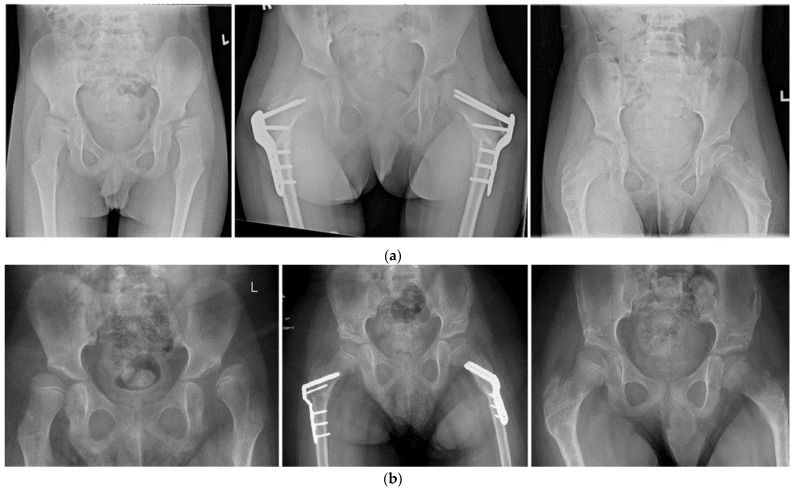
Case examples of hip reconstruction. (a) Case 1: Pediatric Hip Plate. Patient 1 is a boy with CP—GMFCS V spastic quadriplegia. The first radiograph is at age 6 years. At this time, he was scheduled for a bilateral VDRO †. The second radiograph shows postoperative results at 3 months postoperatively. The third radiograph shows the postoperative results at 1 year and 2 months postoperatively (which was 6 weeks after removal of metal). (b) Case 2: Locking Cannulated Blade Plate. Patient 2 is a boy with CP—GMFCS IV spastic diplegia. The first radiograph is at age 10 years. At this time, he was scheduled for a bilateral VDRO and bilateral Dega pelvic osteotomy. The second radiograph shows postoperative results at 6 months postoperatively. The third radiograph shows the postoperative results at 1 year and 2 months postoperatively and 6 weeks after removal of metal. † VDRO Varus derotation osteotomy.
